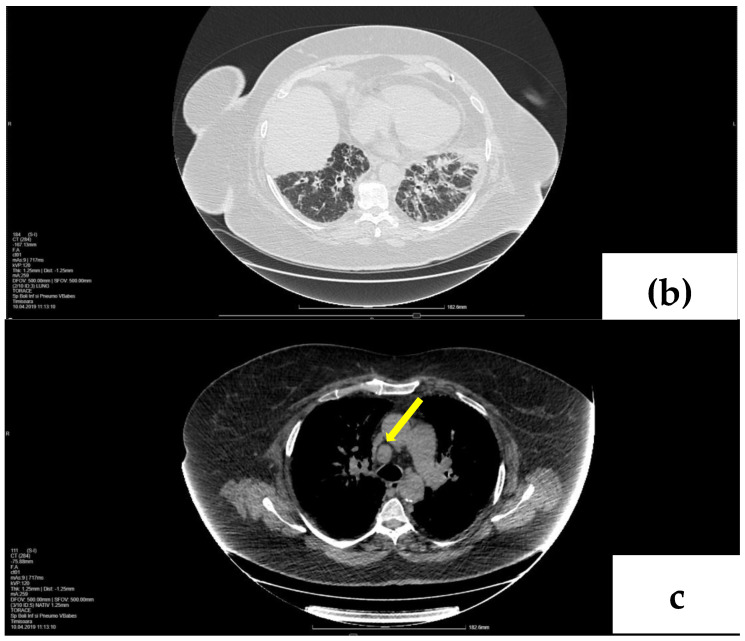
CT scan at the baseline. Axial lung windows at the level of the middle lobe (a) and lower lobes (b) with a dense mass in the right middle lobe (RML), cuffing of bronchovascular bundle with perilymphatic micronodules. (c) Right paratracheal (4R) lymphadenopathy (arrow).
